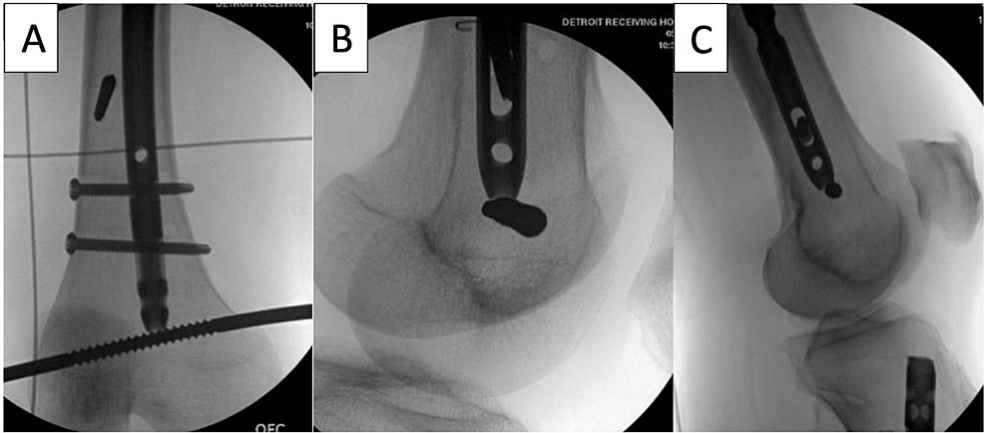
In the case of comminution and a concern for loss of leg length while doing this procedure, a 5.0 mm Schanz pin can be placed perpendicular to the nail abutting the distal tip of the nail as seen on the anterior-posterior (AP) radiograph (A), oblique (B) and lateral (C). This prevents shortening at the femoral fracture site when the distal locking screw is removed. This is a maneuvering pin and not reference pin.
